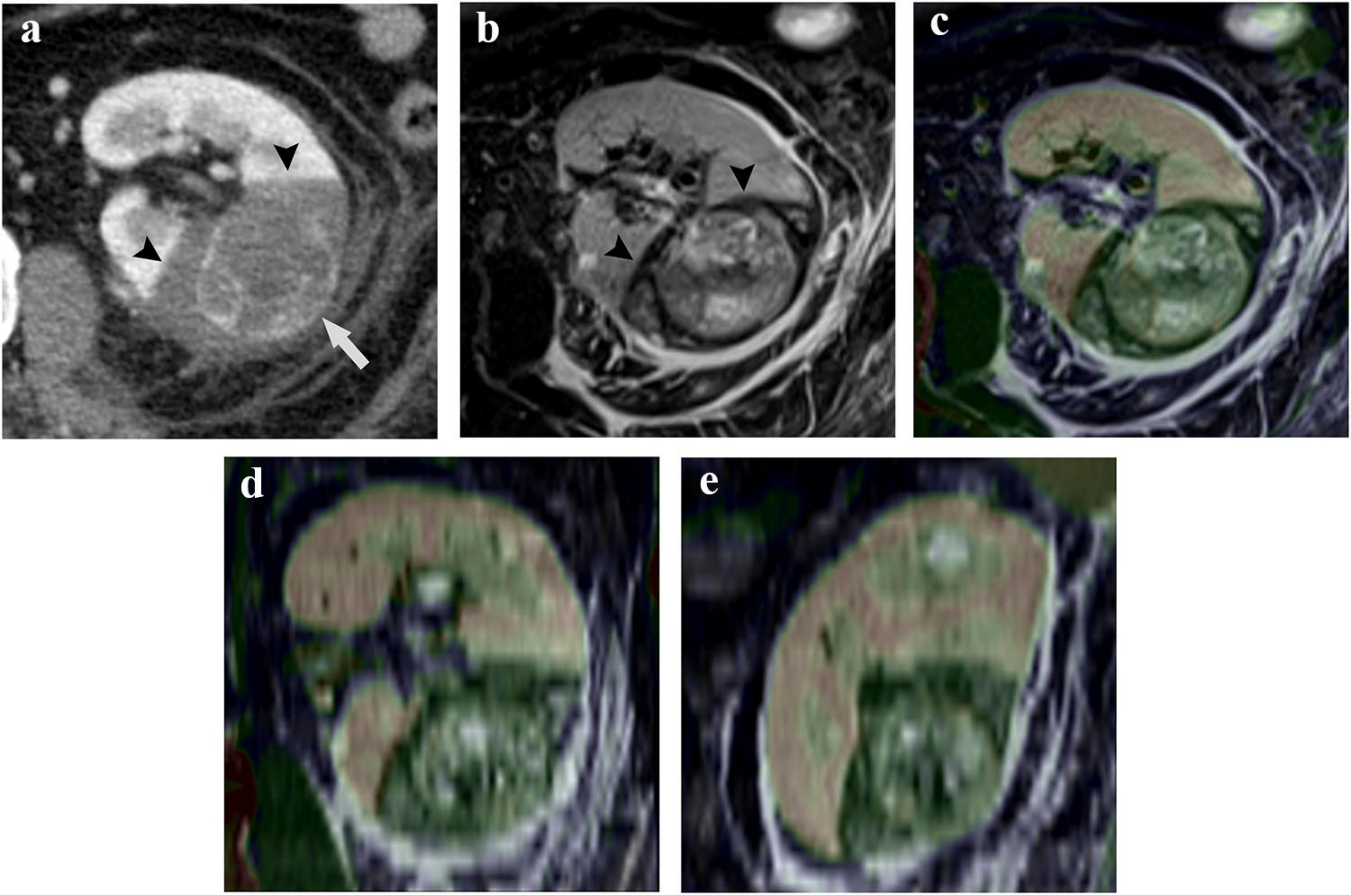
66 year-old man with biopsy-proven clear-cell renal cell carcinoma with a maximum diameters of 4.1 cm who underwent percutaneous cryoablation 2 days ago. A In the nephrogenic phase of dynamic CECT image, the ablation zone is visualized as the unenhanced region (arrowhead) and residual heterogeneous tumor enhancement (arrow) is shown slightly. B In the fat-suppressed T2-weighted MRI image, the ablation zone shows hypointensity (arrowhead) relative to the renal parenchyma. The minimal margin on MRI was 4 mm. C, D, E, Fusion images combining the fat-suppressed T2-weighted MRI image and the nephrogenic phase of dynamic CECT image. Axial (C), coronal (D), and sagittal (E) images of the maximum cross-section of the tumor. The average match score of the two board-certified diagnostic radiologists on a 5-point scale is 4.7 points (radiologist 1 [axial, 5; coronal, 4; sagittal, 4] and radiologist 2 [axial, 5; coronal, 5; sagittal, 5])
